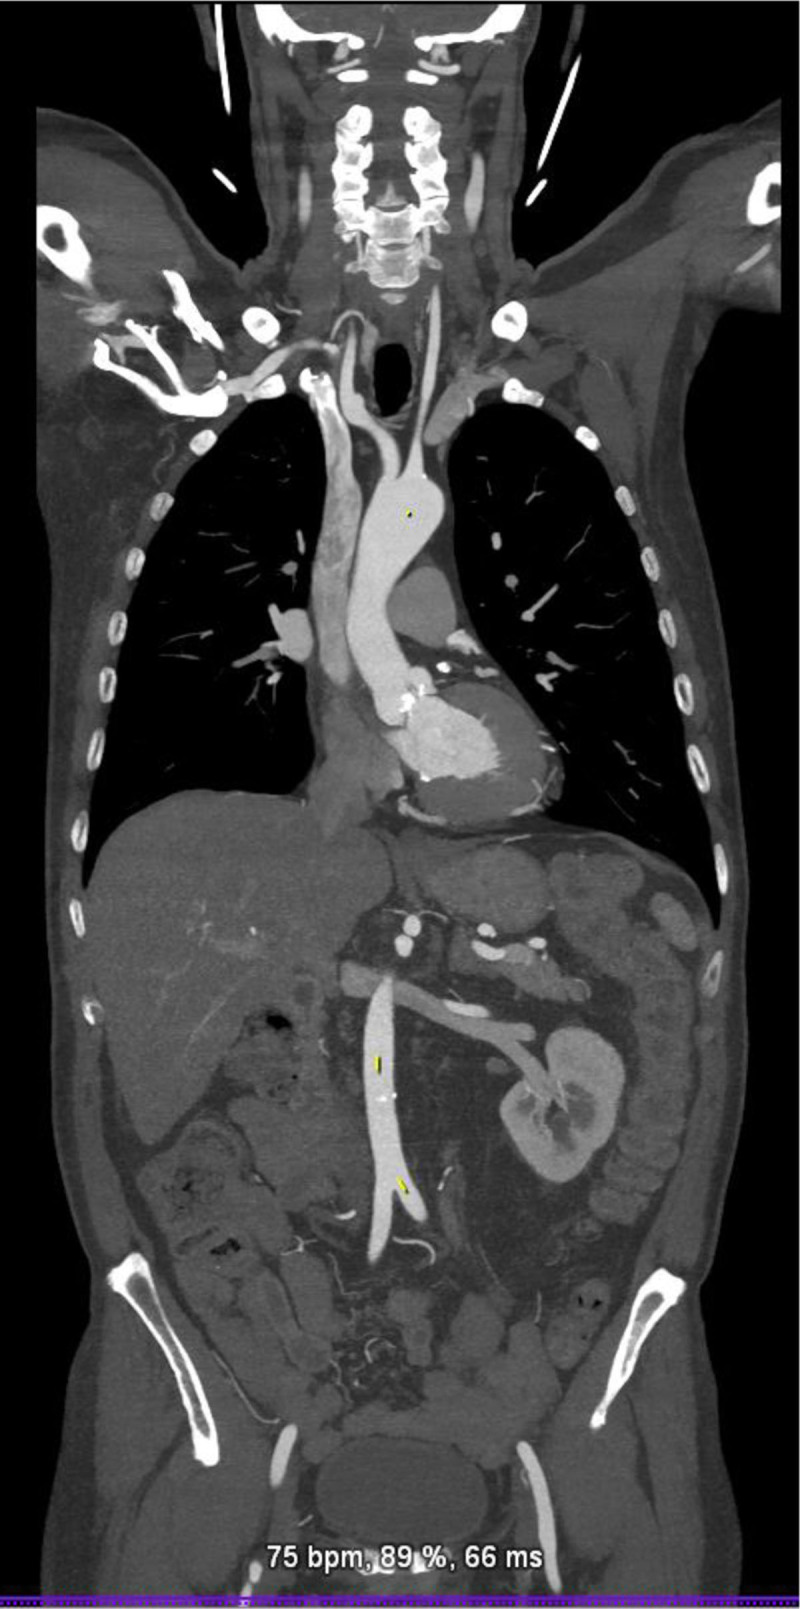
Cardiac computed tomography showing the vascular access from the level of the carotid arteries to superficial femoral arteries.
